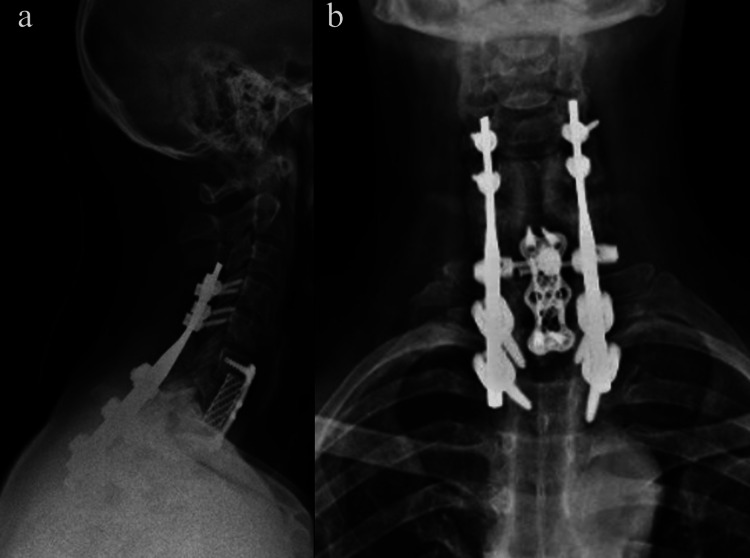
Follow-up anterior-posterior and lateral X-ray images at three months. Follow-up cervical X-ray images showing maintained reduction and alignment, and good fusion.
